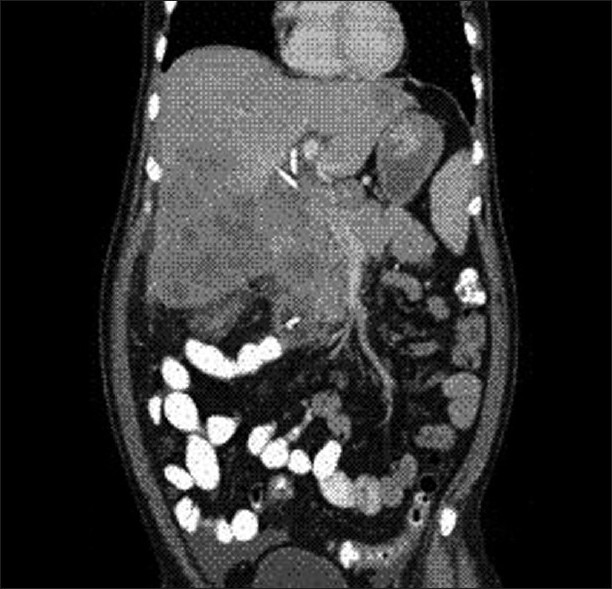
CT abdomen (coronal section) showing a large caudate lobe liver mass involving the gall bladder and common bile duct at porta with intrahepatic biliary radical (IHBR) dilatation
